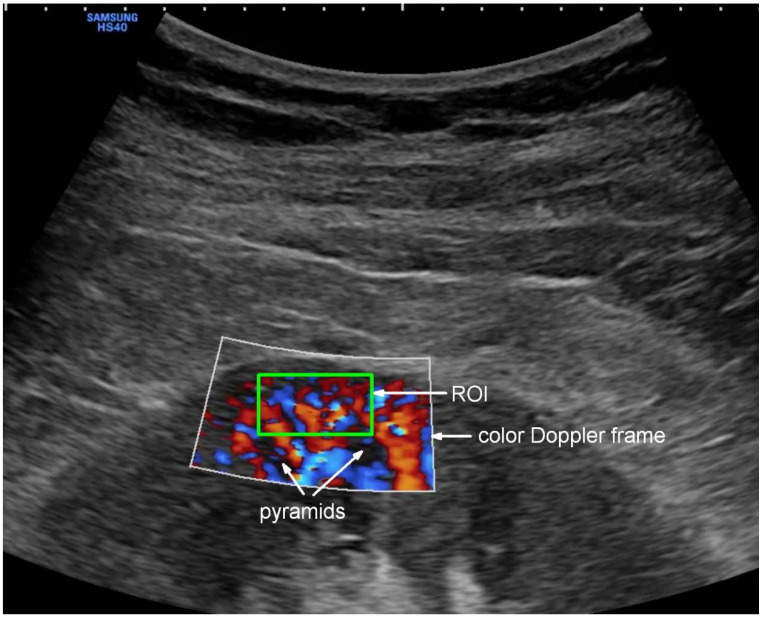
Color Doppler visualization of Renal Cortical Perfusion with selected ROI. The green box shows the location of the region of interest (ROI) for PixelFlux software (v. 18_03_11) flow quantification.
